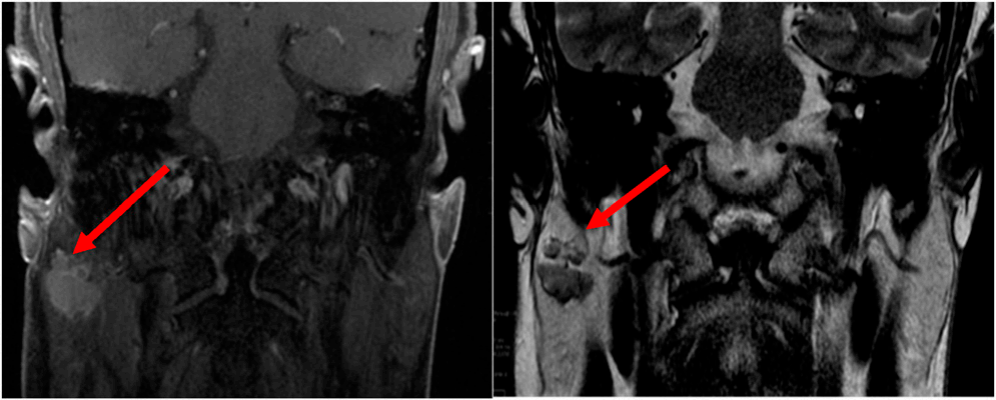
Incidental finding on head MRI with coronal postcontrast fat saturated image of the brain (left) and T2 weighted image of the head (right) demonstrating avidly enhancing bilobed T2 hypointense lesion within the right parotid gland.
